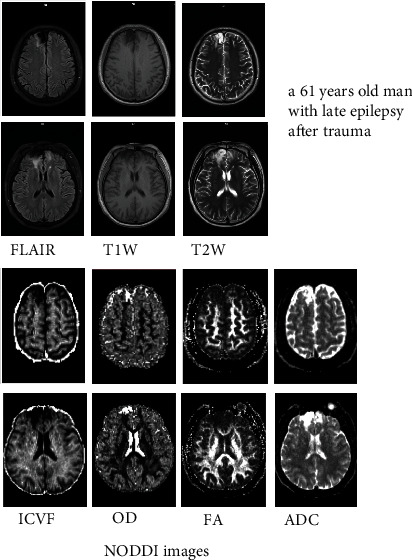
A 61-year-old man had late epilepsy that occurred six months after trauma. He had an encephalomalacia with FLAIR hyperintense lesions in both frontal lobes (FLAIR, T1W and T2W images, and NODDI ICVF, OD, FA, and ADC images).
